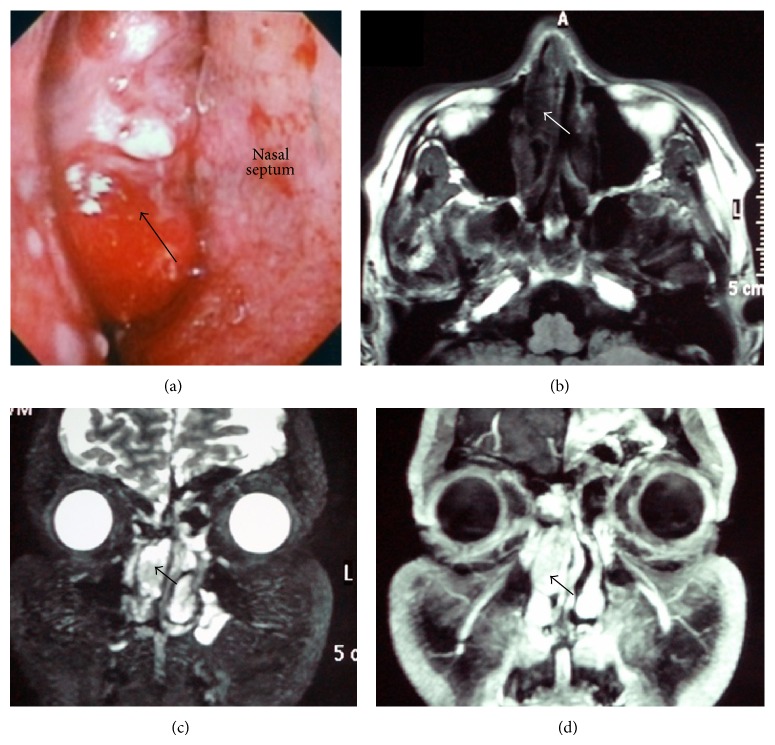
Preoperative local finding and MRI in case 1. (a) Tumor in the right nasal cavity (black arrow). (b) T1-weighted axial image showing low-intensity mass (white arrow). (c) T2-weighted coronal image showing a heterogeneous-intensity mass (black arrow). (d) T1-weighted coronal image (gadolinium+) showing contrast enhancement (black arrow).
